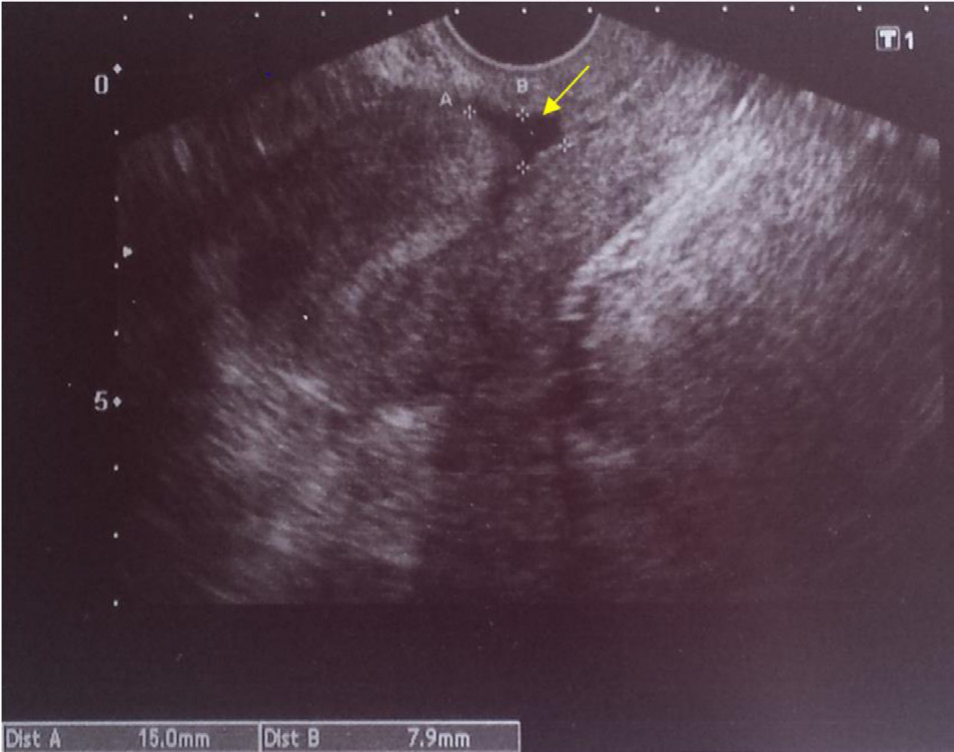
Ultrasound image of an isthmocele. Yellow arrow points towards the isthmocele.
